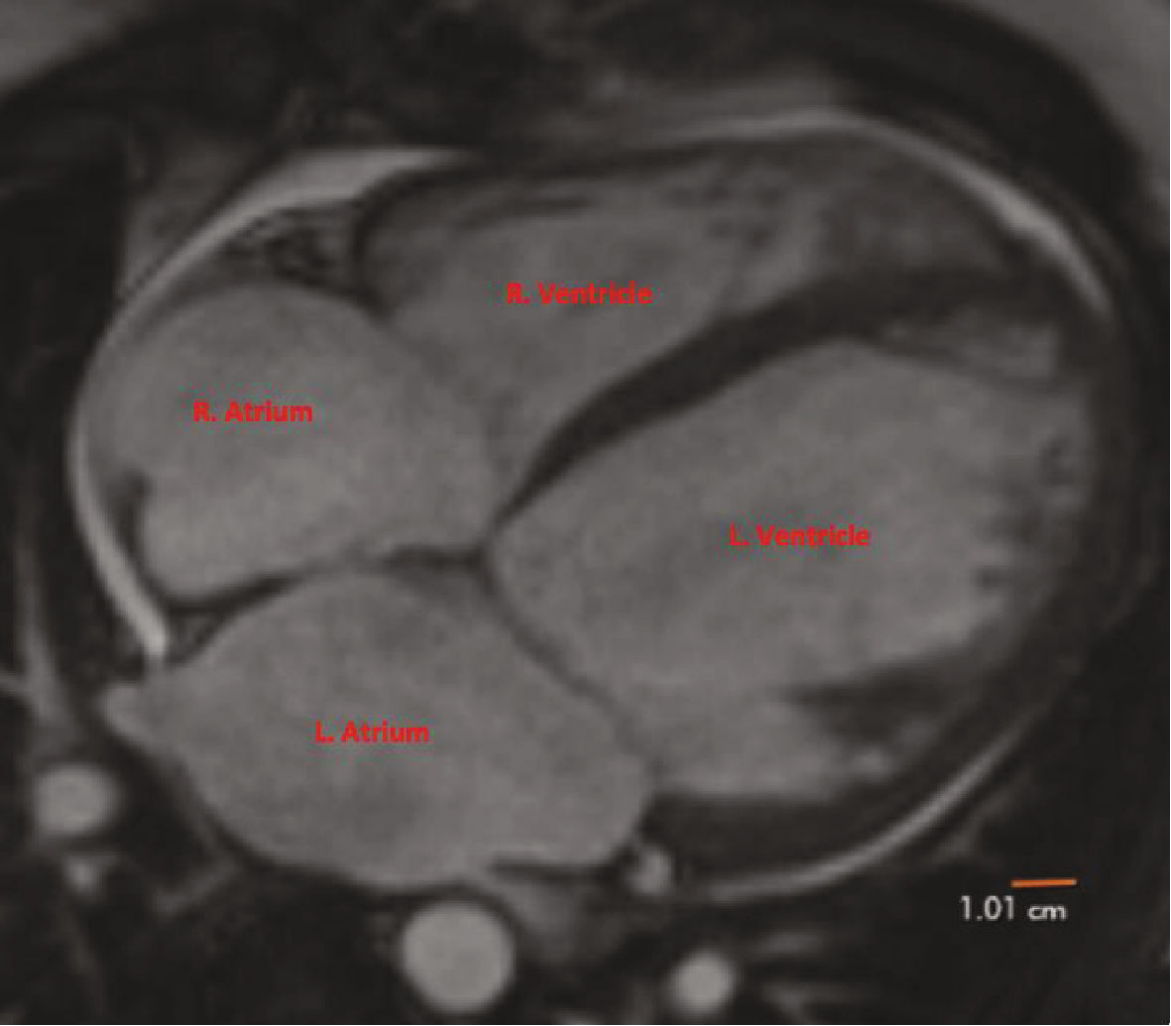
Cardiac MRI showing normal wall thickness and dilated right and left atria and dilated right and left ventricles. The red bar is a standard reference ruler. Markers are placed to indicate the anatomical locations of the ventricles and atria.
